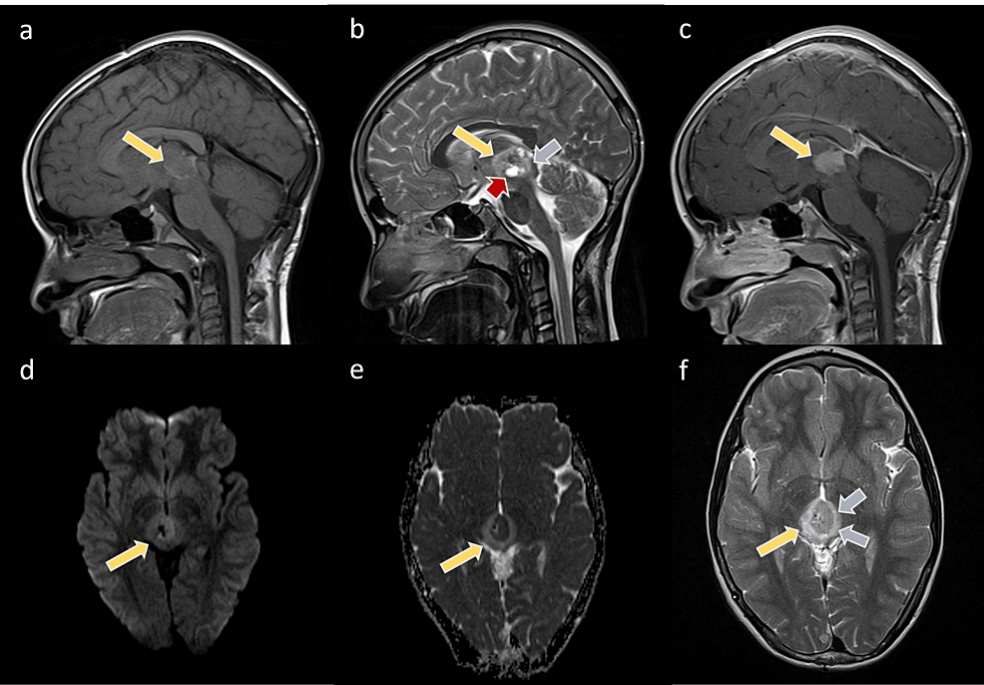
Selected MRIs of the patient after the recurrence of the mass The images show the pineal mass larger than the initial lesion (yellow arrows). The mass shows low to intermediate T1 signal intensity with the bright surrounding rim (a) and heterogeneous low T2 signal intensity (b, f)) with intralesional cysts (red arrow). The mass is enhanced in the post-contrast T1WI sequence (c) and restricted on the DWI (d,e). Noted is the surrounding edema (blue arrows) of the bilateral thalami and tectum on the T2WI.
